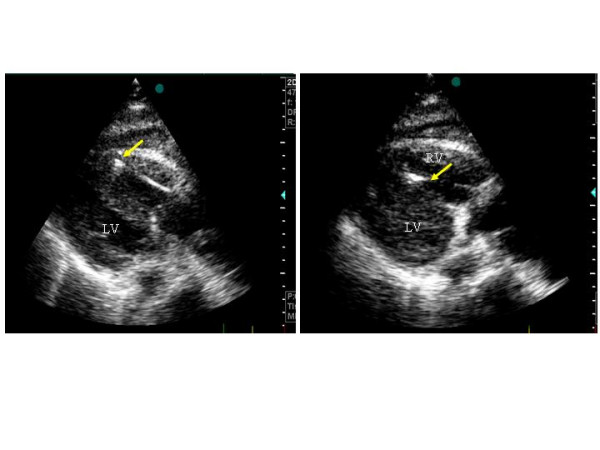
Use of 2-D echocardiography for monitoring the performance of endomyocardial biopsy in a HT recipient. The arrow indicates the site of the biopsy. Left panel: at the apex of right ventricle, right panel: al the level of the right side of the interventricular septum.
